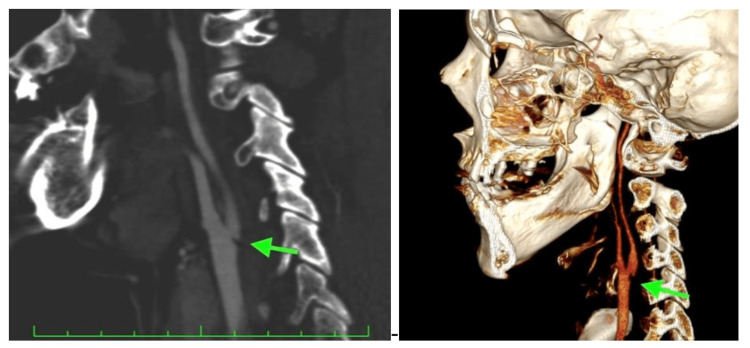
Computed tomography angiography (CTA) reveals a localized membrane-filling defect (green arrow)
